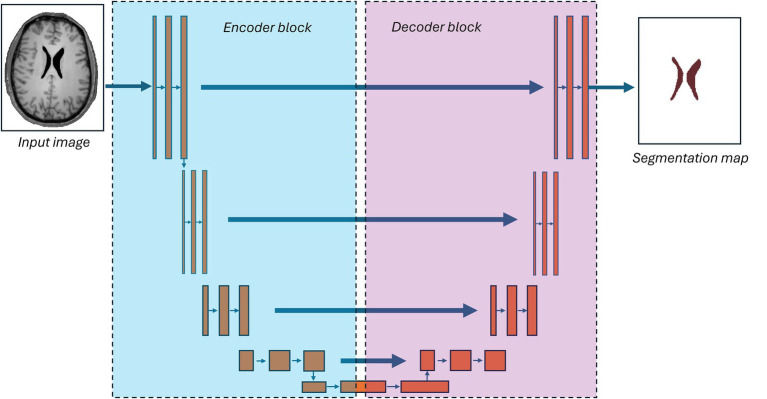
From an input MR image, a U-net model sends the image into a low-dimensional embedding (“the encoder”) then subsequently recovers the image segmentation map (“the decoder”).
